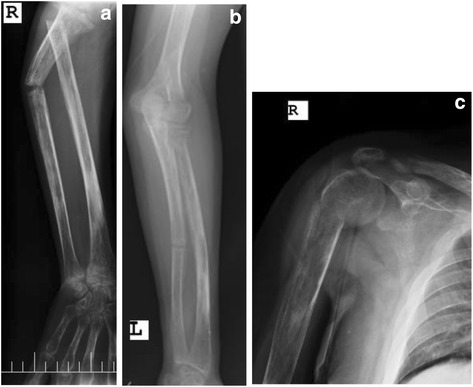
X-Ray radiographs showing severe osteomalacia and multiple fractures. a Right radial head and right ulnar shaft fracture; b Left mid ulnar shaft fracture; c Right humerus anatomical neck fracture and Distal clavicular resorption
